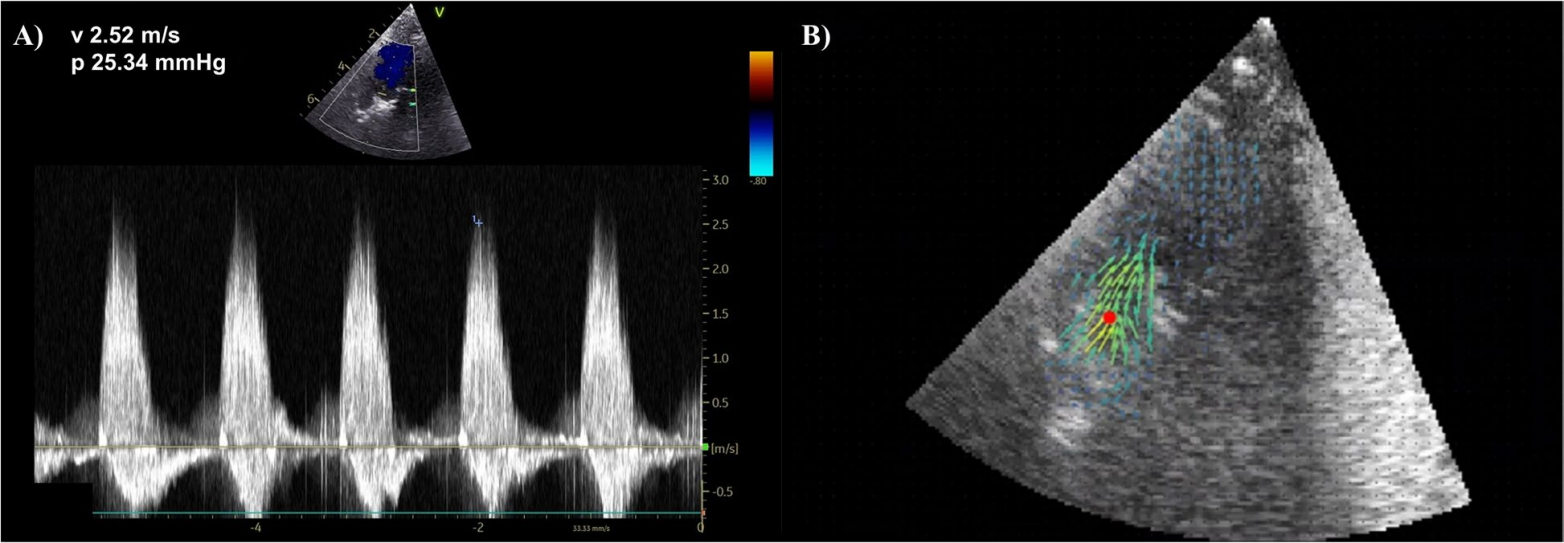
A Example continuous wave Doppler acquisition at 250 mL/s flow rate with manual measurement shown. B Example BSI velocity vector interface, showing the velocity of flow through the region of interest
